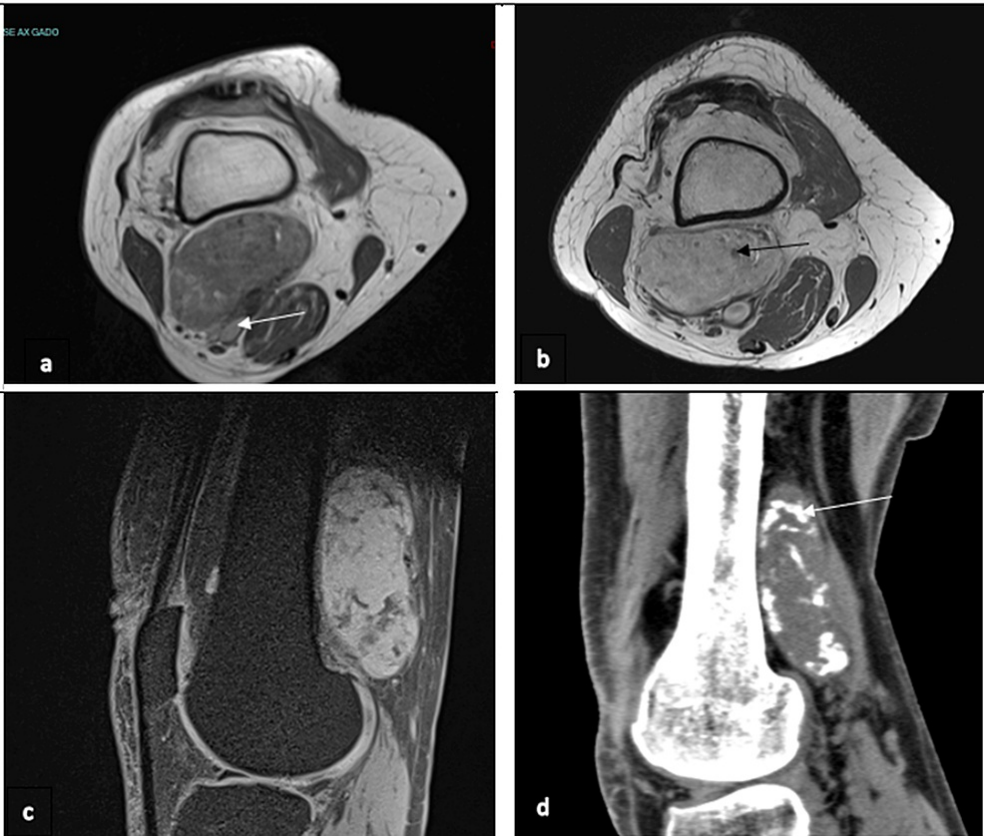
Imaging of the lesion at diagnosis a. Preoperative MRI  with axial T1-weighted image with the lesion adjacent to the popliteal pedicle (white arrow); b. Axial T1-weighted gadolinium-enhanced image, showing calcifications (black arrow); c. Sagittal T1-weighted fat-saturated image after gadolinium injection shows heterogeneous uptake, with some areas of low signal intensity indicating calcification; d. Sagittal CT scan demonstrating calcifications of the lesion (white arrow)
